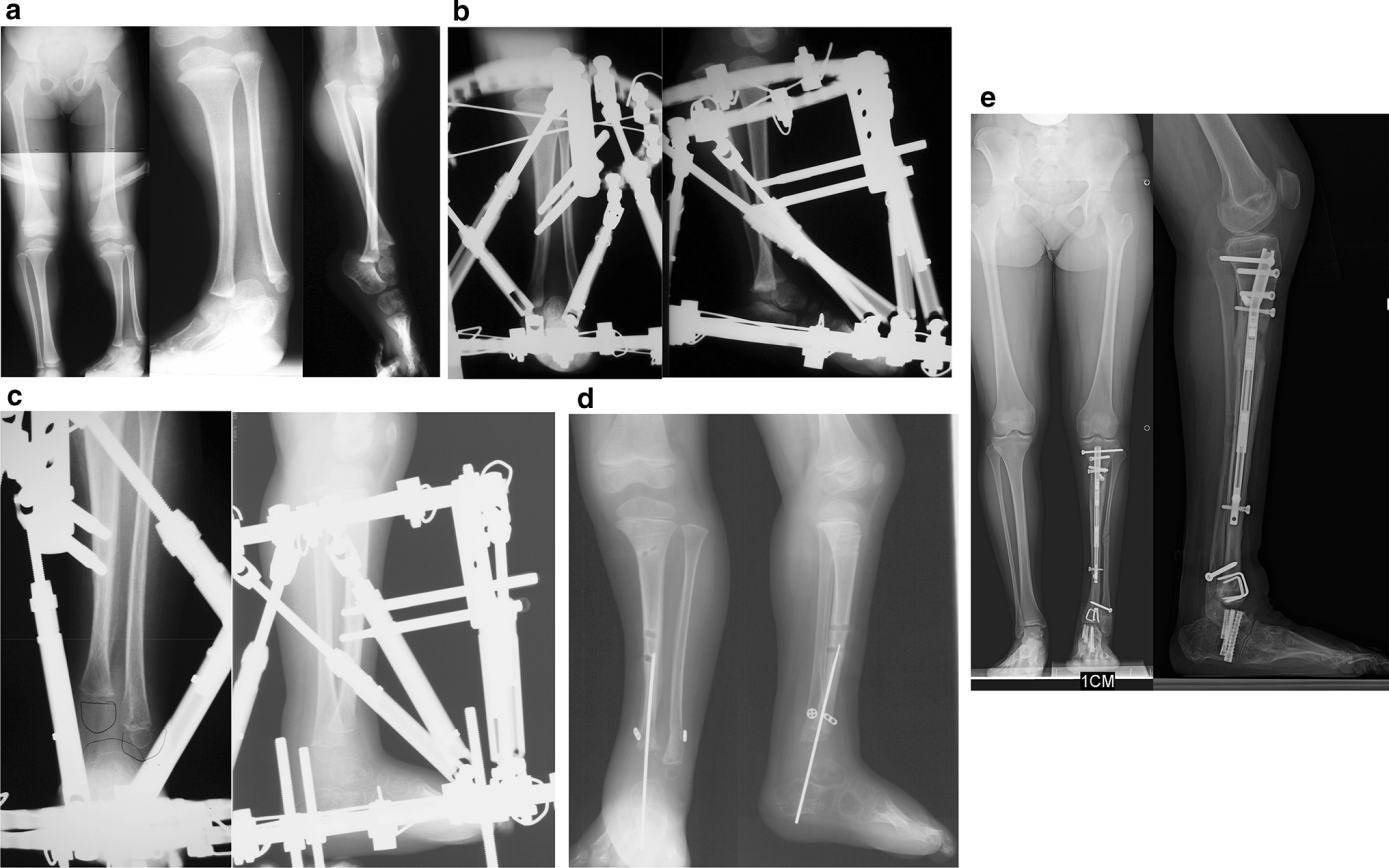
a Standing (*left*), mortis view (*middle*) and lateral view (*right*) radiographs of a 2-year-old girl with Paley type 3A unilateral tibial hemimelia. The diastasis of the distal tibia and fibula with the talus in between is very evident. The talus always remains in line with the distal fibula and together they internally rotate around the distal tibia. The talus is shortened relative to the distal tibia but not relative to the distal fibula. There is relative overgrowth with proximal migration of the fibula. There is a mild distal tibial varus diaphyseal bowing. b AP (*left*) and lateral (*right*) radiographs showing TSF applied to the tibia and foot. There is no fixation in the fibula or talus. More current constructs would fix to these as described in the text. The foot is in internal rotation and equinus. c AP (*left*) and lateral (*right*) radiographs after gradual correction using TSF. The talus is under the distal tibial epiphysis and the foot and fibula have rotated externally relative to the tibia. There is no longer any tibio-fibular diastasis. The equinus deformity is also corrected. This gradual correction took 12 weeks. d AP (*left*) and lateral (*right*) radiographs after removal of the external fixator 3 months following open ankle reconstruction. The ankle reconstruction included a biologic arthroplasty to create a concave surface on the distal tibial epiphysis that is congruent to the talar dome convexity. The ankle joint was pinned with an axial wire and the diastasis of the tibia and fibula were fixed with a suture-washer syndesmotic repair (TightRope®, Arthrex, Naples, FL). The axial wire was left in place for another 3 months to prevent recurrent deformity at the ankle. Note the proximal fibula was brought down to station. e AP (*left*) and lateral (*right*) radiographs at age 14 years after she underwent two successful lengthenings; one external fixator lengthening at age 6, and one implantable nail lengthening at age 14 (Precice™; NuVasive Inc., San Diego, CA). She also had a supramalleolar and subtalar osteotomy. Note the ankle joint is stable and well preserved. The ankle has about 20° of motion. The proximal fibula is at station. Most of this hardware was removed at a later date. Due to the limited ankle motion, the leg lengths were intentionally corrected to leave a 1-cm difference
